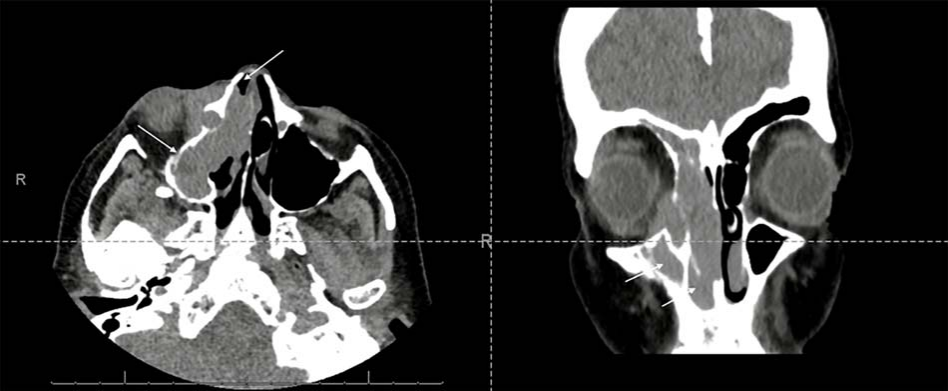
Axial and coronal CT sinus images showing an extensive soft tissue mass within the right maxillary sinus which completely obliterates the sinus cavity and extends into the right nasal cavity (arrows). It also involves the right ethmoid and frontal sinuses. The mass extended through the lamina papyracea into the right orbit, abutting and displacing the globe, and is seen to invade the nasolacrimal system.
